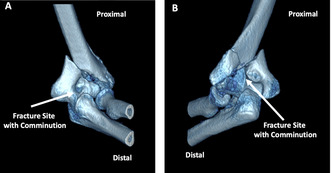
Computed tomography. Three‐dimensional reconstruction computed tomography scan of the left elbow (A,B) showing significant comminution of the articular block of the distal humerus as well as intra‐articular involvement.
